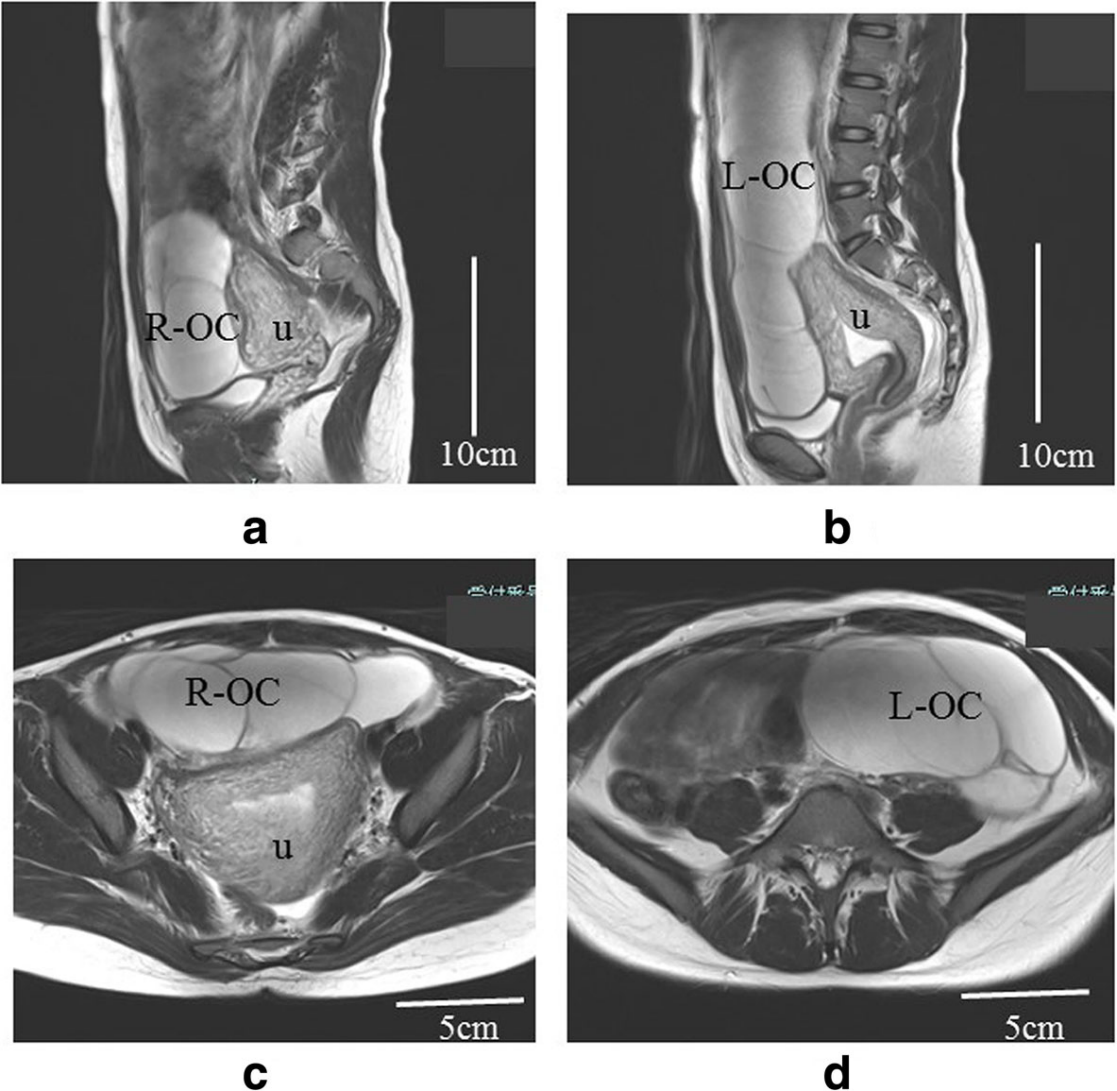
Magnetic resonance imaging scans of the ovarian cysts on postoperative day 24. a Sagittal view of the right ovarian cyst (R-OC). b Sagittal view of the left ovarian cyst (L-OC). c Transverse view of the R-OC. d Transverse view of the L-OC. u, uterus
